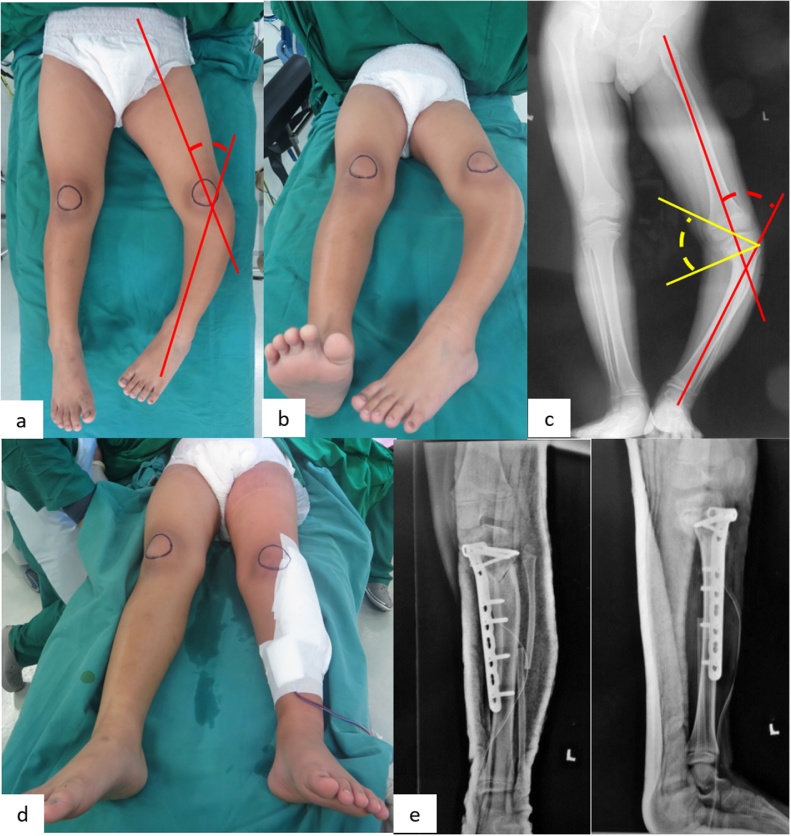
Pre and post operative results. (a) Pre operative clinical picture. Tibiofemoral lines from clinical picture was 46°, (b) Pre-operative clinical picture. Birds view, (c) Pre-operative Xray. Red lines – TFA 46°, Yellow lines – Drennan angle 43°, (d) Post operative clinical picture. Tibiofemoral lines from clinical picture was 10°, (e) Postoperative birds view with the deformity already corrected, (f) Postoperative TFA was 10°, Drennan angle was 4.3°, and from lateral xray procurvatum was corrected.
